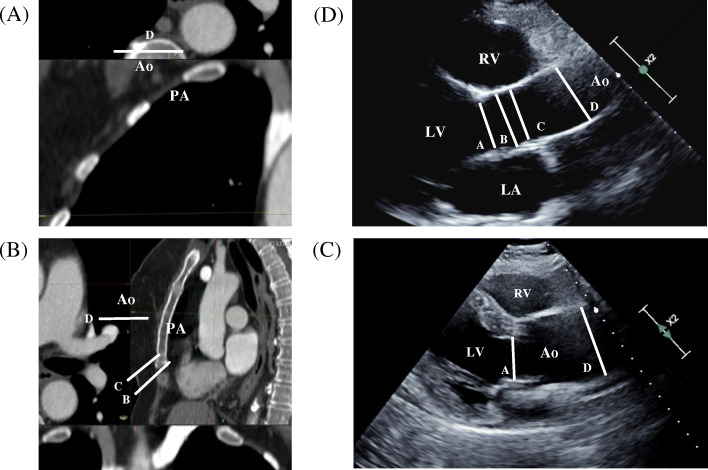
Cardiovascular imaging of the ascending aorta by (a and b) computed-tomography and by (c and d) 2D-transthoracic echocardiography. Aortic annulus (D diameter). Sinuses of Valsalva (B diameter). Sinotubular junction (C diameter). Proximal (tubular) ascending aorta (D diameter). PA, pulmonary artery. Ao, Aorta. RV, right ventricle. LV, left ventricle
